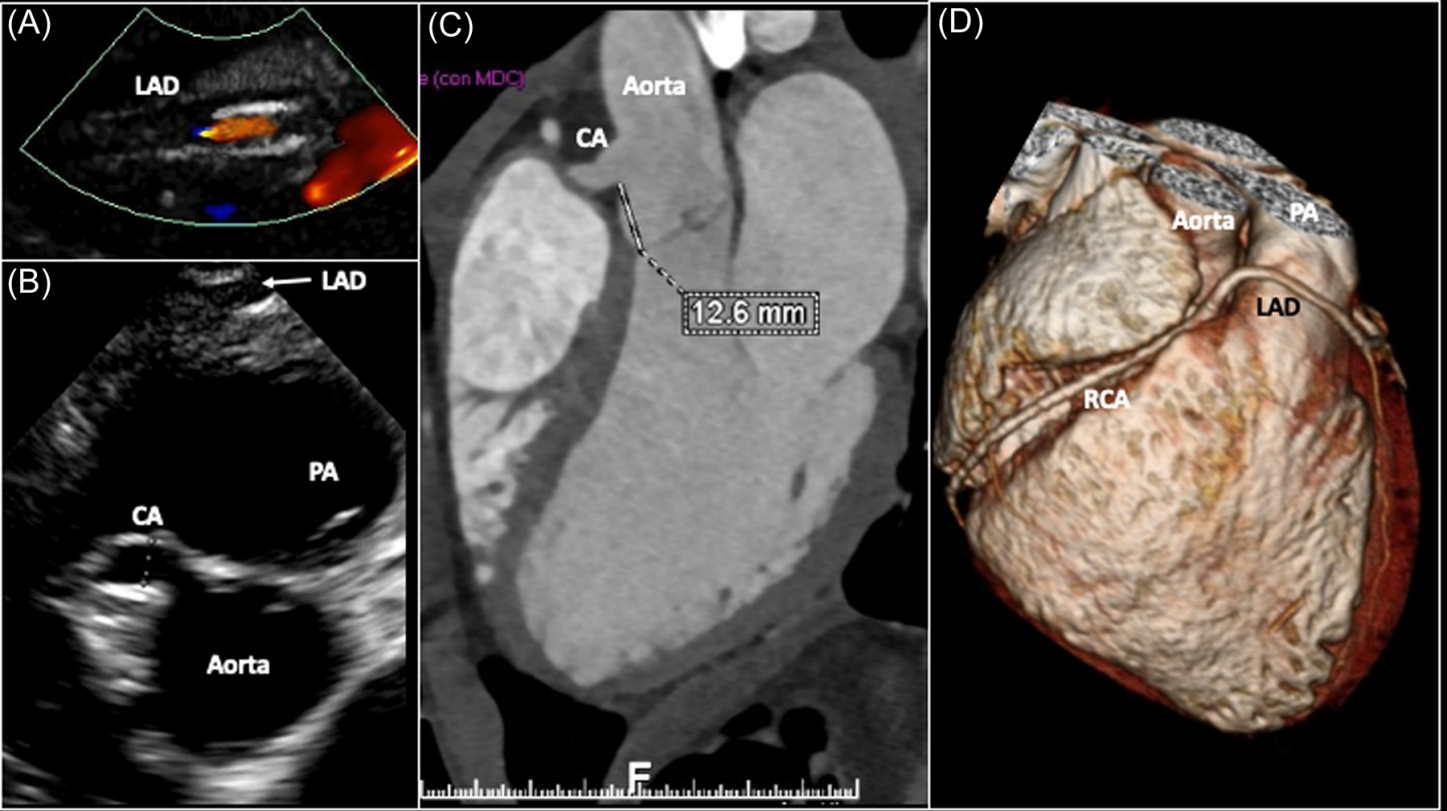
Thirteen year old boy, with a cardiac murmur at the preparticipation screening for competitive swimming. The transthoracic echocardiogram (A and B) showed a dilated coronary artery (transverse diameter 6 mm) originating from the right sinus of Valsalva, with no vessel arising from the left sinus. Anteriorly to the pulmonary artery a vessel can be observed, consisting of the left anterior descending coronary artery. In panel (A), a magnification of this vessel is shown, with color flow Doppler diastolic signal within the vessel. The coronary computed tomography angiogram (C and D) showed a unique coronary artery originating from the right sinus of Valsalva, with the left anterior descending artery originating from the unique coronary artery and running anteriorly at the pulmonary artery. CA, coronary artery; LAD, left anterior descending artery; PA, pulmonary artery; RCA, right coronary artery.
